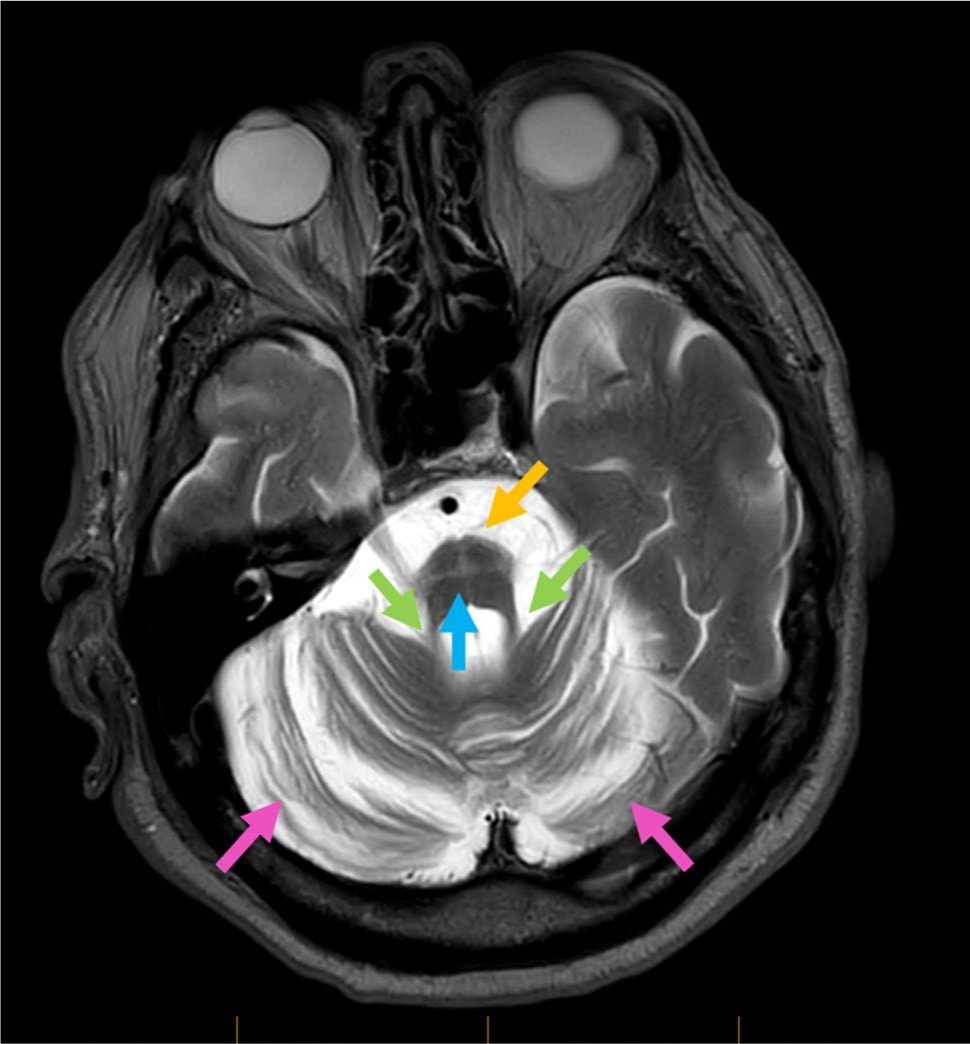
Brain MRI image of proband II showing marked atrophy of pons (yellow arrow), cerebellar peduncles (green arrows), and bilateral cerebellar hemispheres (pink arrows) with an enhanced cruciform signal on T2 and FLAIR sequence in pons, also referred to as hot cross bun sign (cyan arrow)
